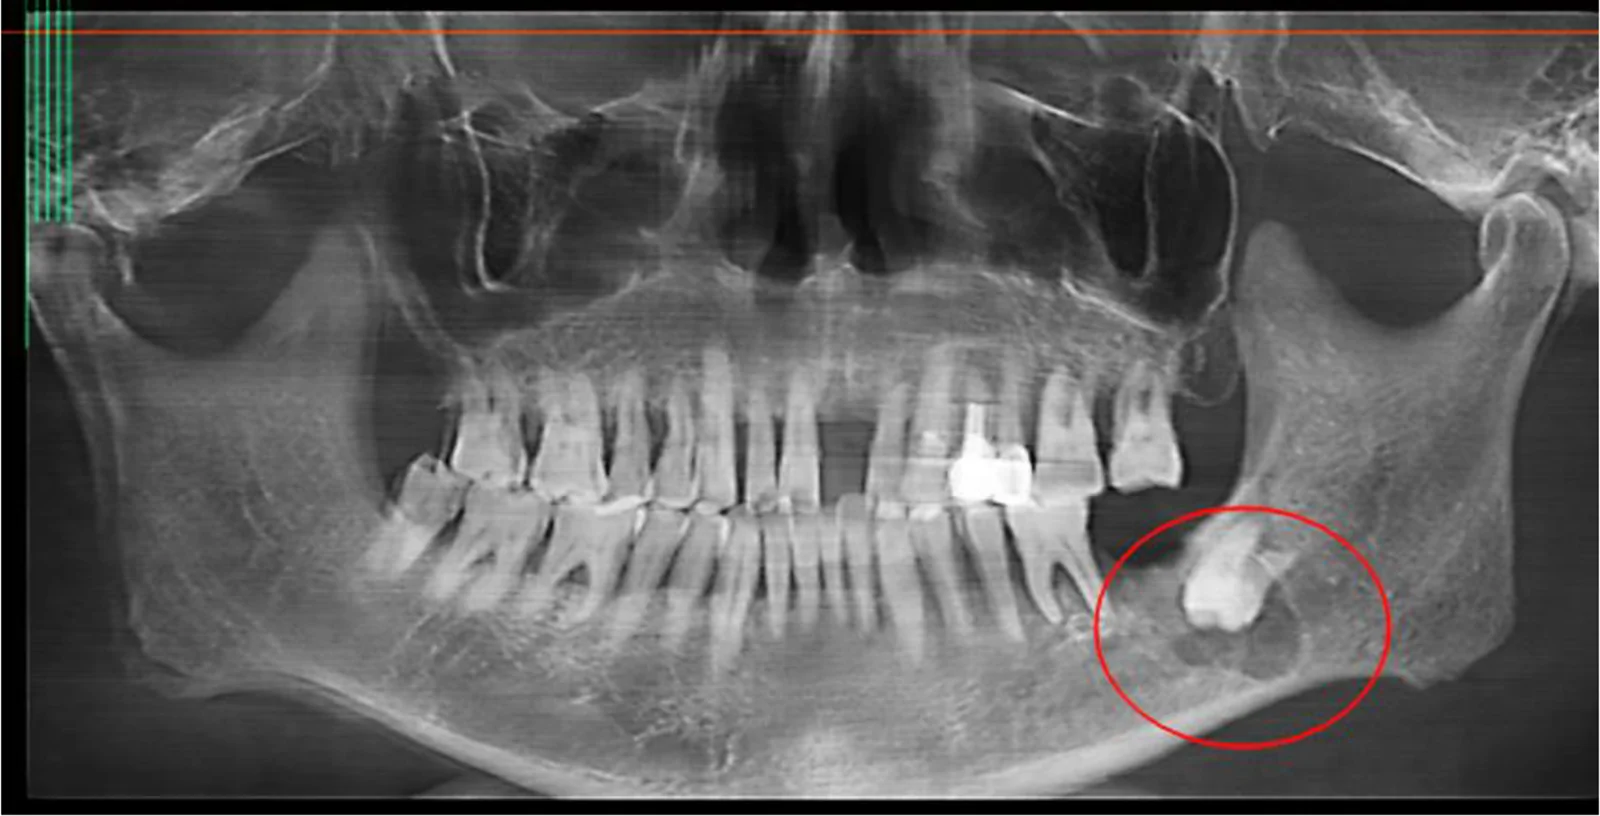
CBCT scan showing a well-circumscribed, unilocular radiolucency with defined borders, enveloping part of tooth 38. Tooth 37 was previously extracted. CBCT = cone-beam computed tomography.
